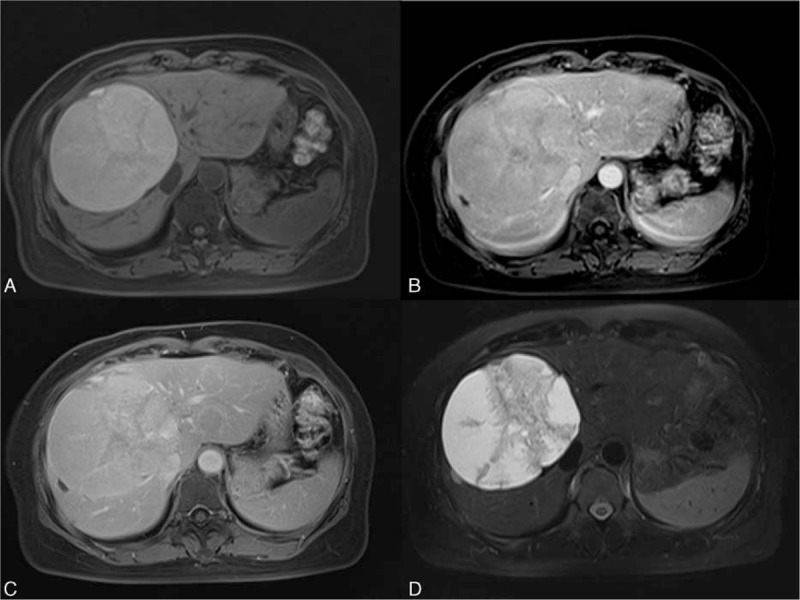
A 50-year-old woman with a mucinous cystic neoplasm (MCN). MCN with low-grade dysplasia was diagnosed after surgical resection. A, T1 precontrast image shows a hyperintense mass with some internal septation at the right lobe of the liver. The T1 high signal is due to high proteinaceous and colloid contents. The fluid-containing parts show no enhancement after contrast on T1-weighted images in the arterial (B) and the later phases (C). D, T2-weighted image with fat suppression depicts the fluid-containing parts with high signal. Note the septations are also clearly identified on T2WI.
